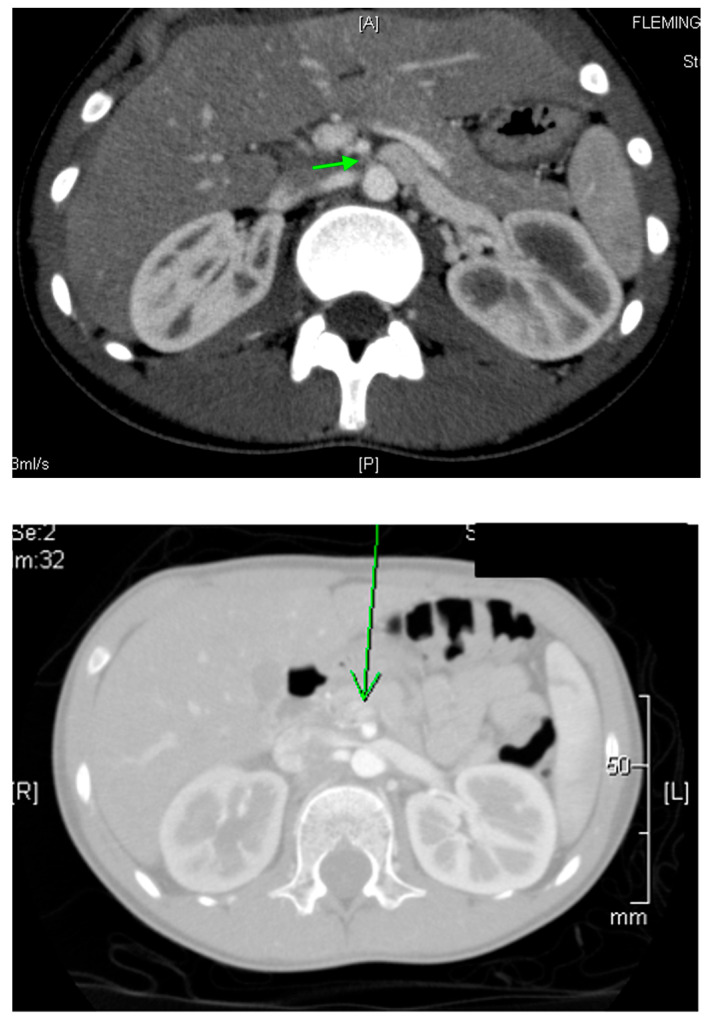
CT with angiography shows the compression of the left renal vein between the aorta and the proximal superior mesenteric artery as indicated by green arrows (Nutcracker Syndrome).
